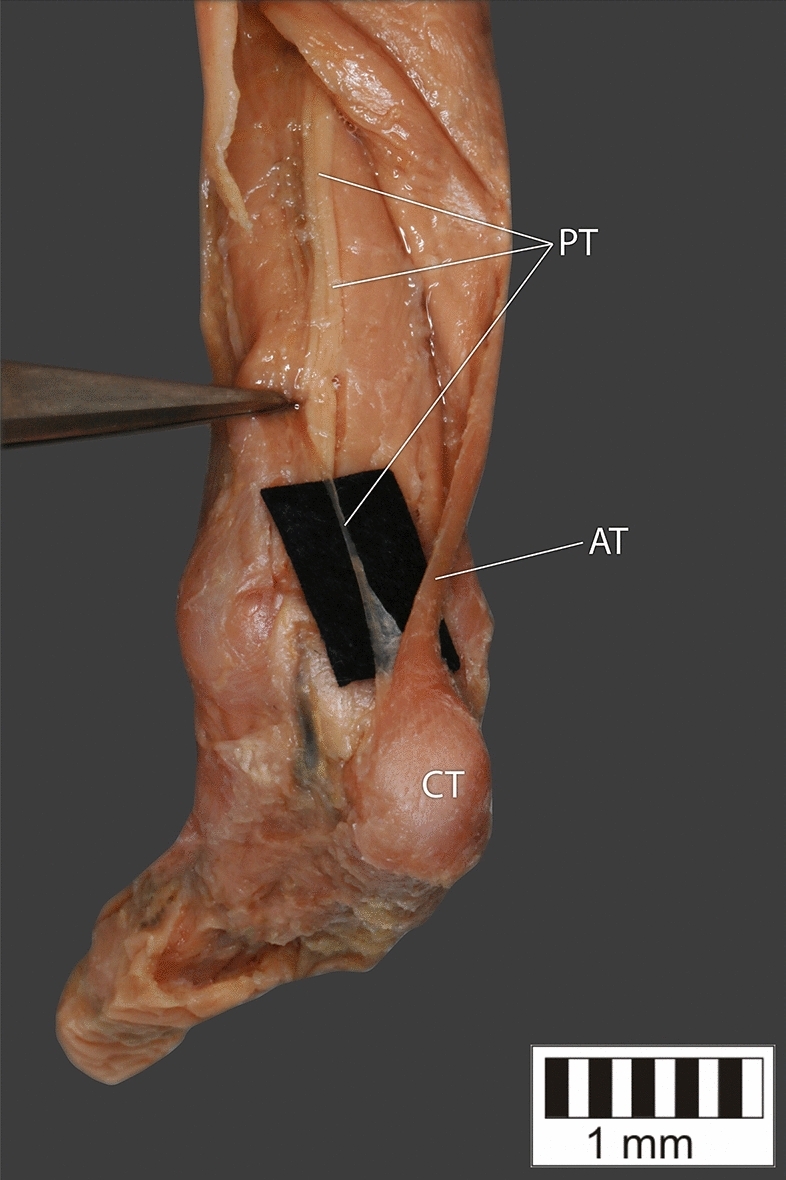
Type IV of plantaris tendon. *CT* calcaneal tuberostity, *AT* Achilles tendon, *PT* plantaris tendon.
